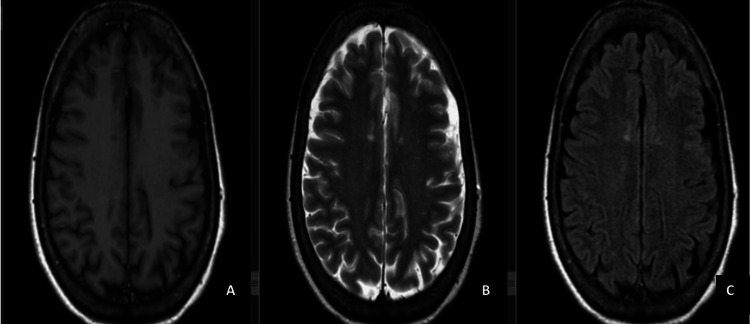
Cranial MRI with T1W (A) and T2W/FLAIR (B, C) hyperintense signals in the left occipital and bilateral temporal convexities. Nodular and patchy areas of T2W/FLAIR hyperintense signals in the centrum semiovale, periventricular white matter, cerebral peduncle, and pons. Small CSF-like signals in the medial temporal lobes. A: T1W. B: T2W. C: FLAIR. T1W: T1-weighted; T2: T2-weighted; FLAIR: fluid-attenuated inversion recovery; CSF: cerebrospinal fluid
